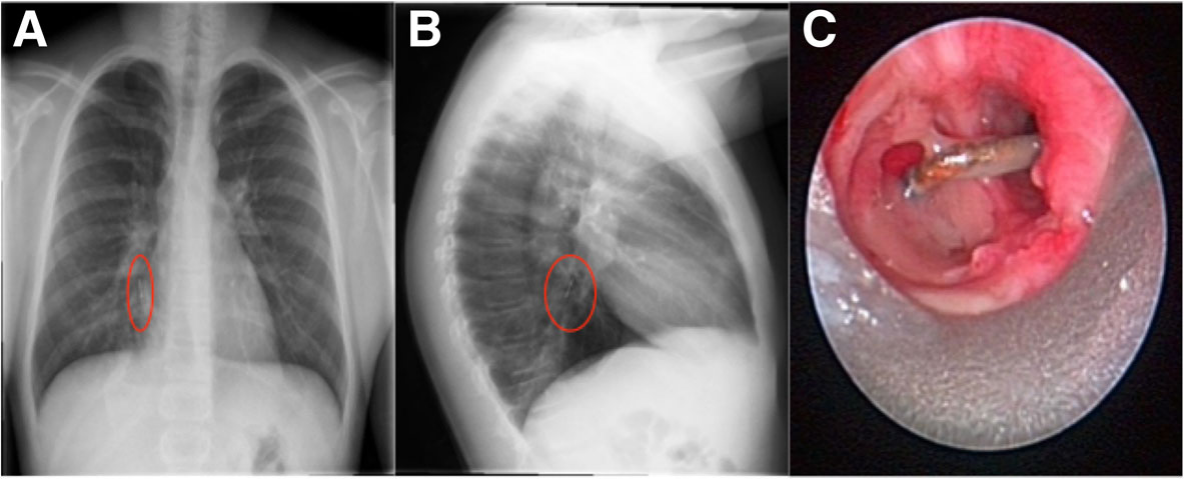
Case 2 - Posteroanterior (PA) chest x-ray showing metallic foreign body in right lower lobe bronchus (a). Lateral chest x-ray shows a metallic foreign body and surrounding consolidation (b). Endoscopic view of an impacted metallic foreign body with surrounding granulation tissue and inflammation (c)
